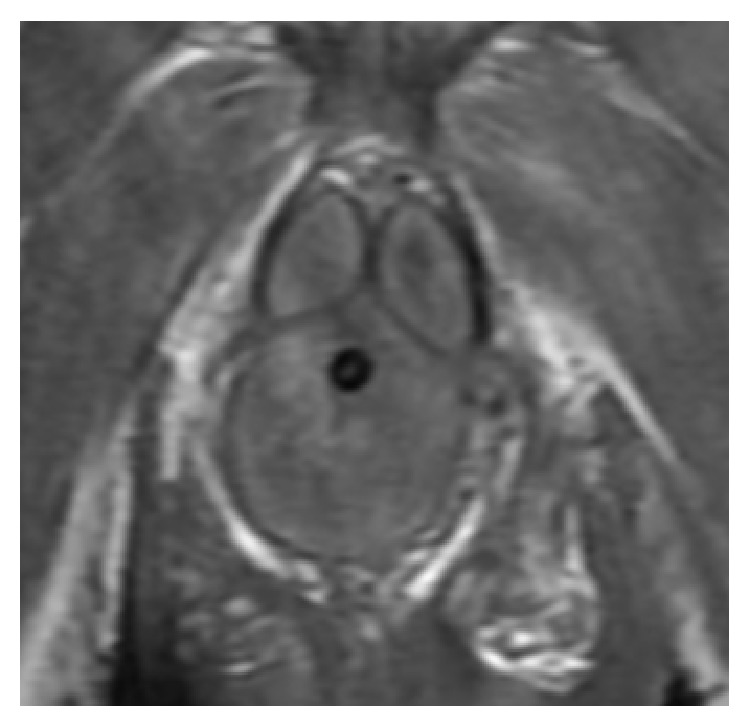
A representative MRI T1 image without gadolinium showing a coronal cut of the base of the penis with both corpora cavernosa and the corpus spongiosum having similar signal intensity.
